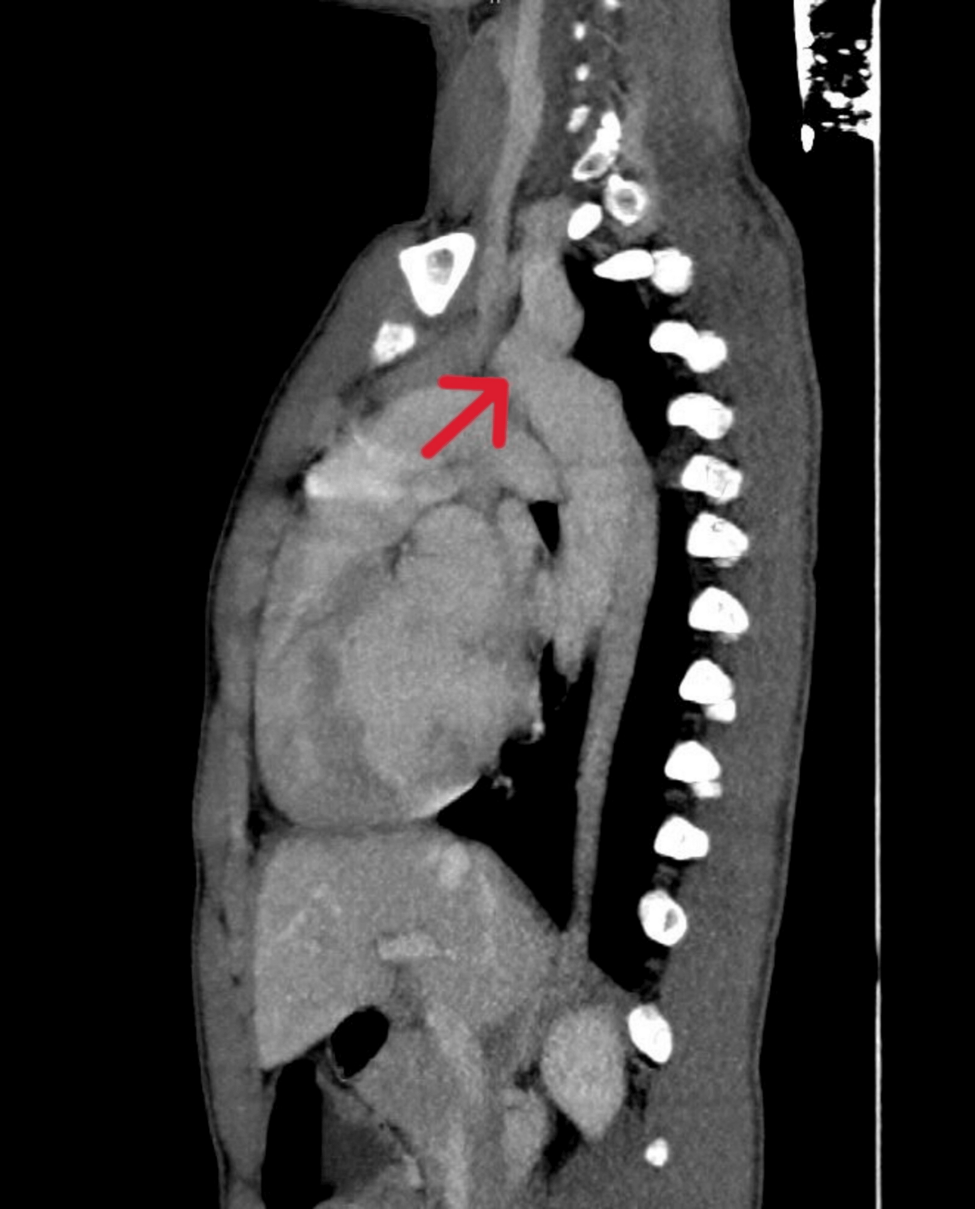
Computed tomography of chest showing the coarctation of aorta (red arrow).
